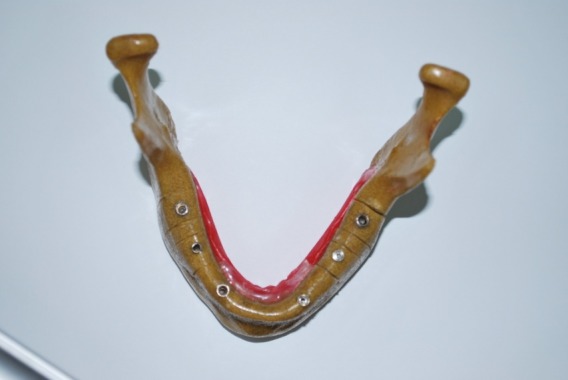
The first series of scans were carried out by NewTom VG x-ray machine in the Department of Oral and Maxillofacial Radiology, Faculty of Dentistry, Tabriz University of Medical Sciences. This x-ray machine uses a cone x-ray beam with 1920×1536 pixel flat panel detector, a rotation of 360º, a pixel size of 0.127 μm and 120 kVp. The scans were carried out at kVp110 kVp, exposure time of 3.6 seconds and 4.71 mA. Initial and final reconstruction was carried out by NNT Viewer software version 2.21 (Quantitative Radiology, Verona, Italy).
